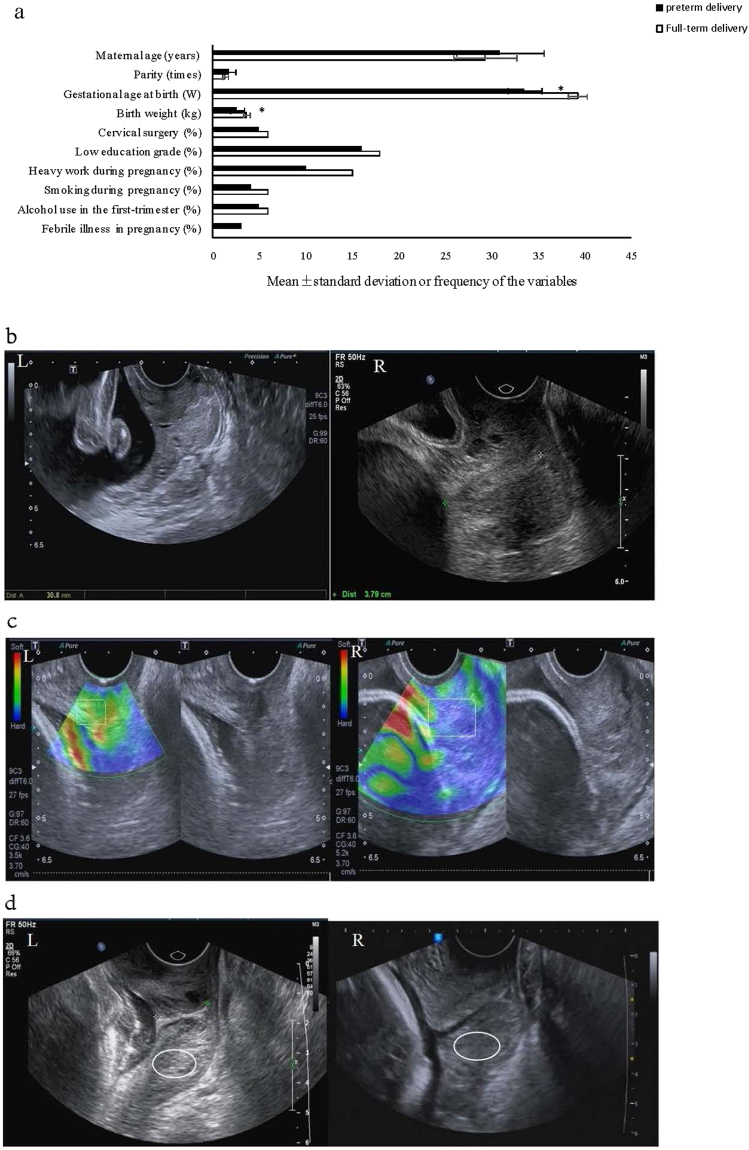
Characteristics of the pregnant patients. Note: (a) Basic characteristics of patients with preterm birth and full-term delivery. P* < 0.05 indicates a significant difference between preterm delivery and full-term delivery; (b) Cervical length as determined by transvaginal ultrasound in pregnant patients. Left (L) shows a shortened cervix (30.8 mm), Right (R) shows a normal cervical length (37.9 mm); (c) Blue area for cervical tissue as determined by sonoelastography in pregnant patients, the blue area indicates the stiffness of the cervical tissue. Left shows the percentage of blue area as an ROI (10.8%) that indicates a soft elasticity of the cervix, Right shows the percentage of blue area as an ROI (29.7%) that indicates relatively stiff cervical tissue; (d) Mean gray value of the sagittal transvaginal view in pregnant patients. Left shows a decreased echogenicity and grayscale value of 4.85, Right shows a normal echogenicity and grayscale value of 11.78.
